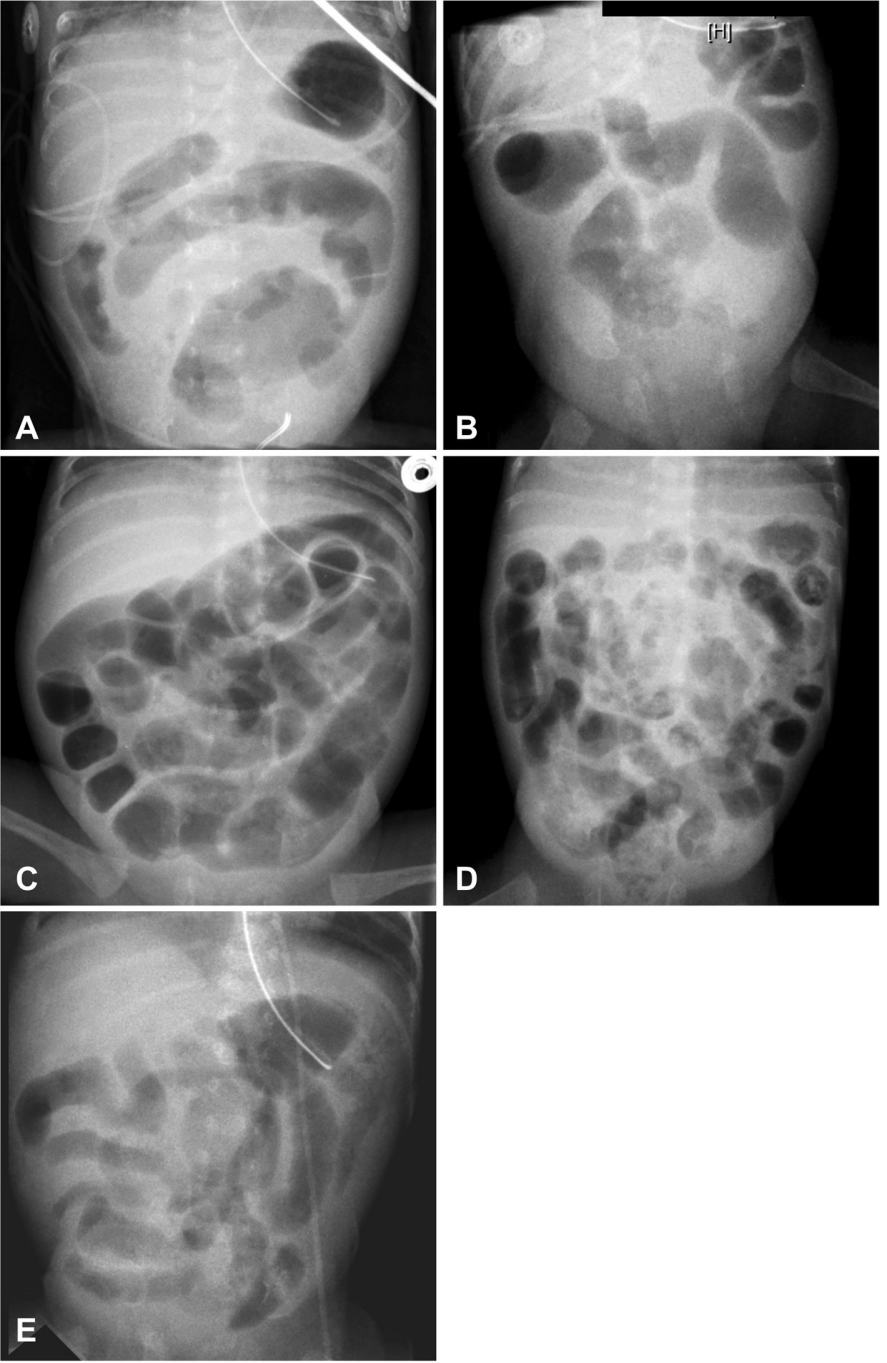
Abdominal X-rays at clinical presentation of volvulus. Abdominal X-rays at clinical presentation of volvulus: Panel A shows possibly suggestive concentric alignement of bowel loops, Panels A, B, E display unspecific intestinal wall thickening, Panel D may well have been compatible with necrotising enterocolitis and Panel C is unspecific.
